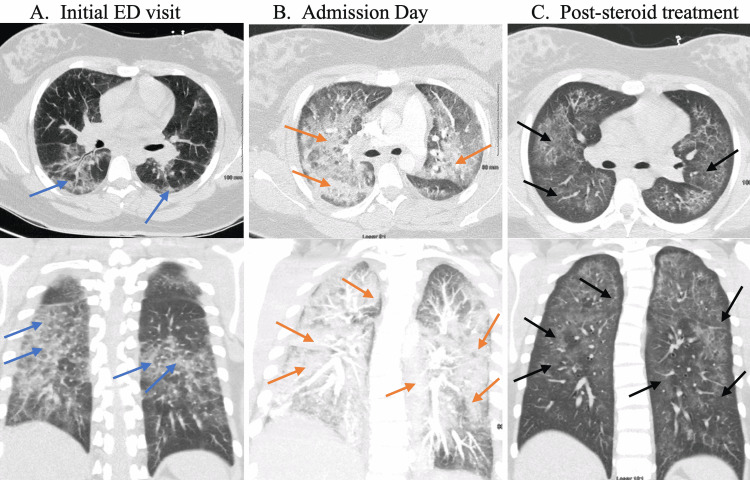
Computed tomography findings A. Initial ED visit: Ground-glass infiltrates in multiple areas in the bilateral upper and lower lobes, along with some regions of peri-bronchial thickening and some infiltrate radiating from the hila (blue arrows). B. Admission day: Worsening bilateral coalescent alveolar infiltrates (red arrows) compared to the initial visit. C. Post-steroid treatment: Marked decrease in the infiltrates (black arrows) 48-hour post-high-dose intravenous steroid treatment. ED: emergency department
